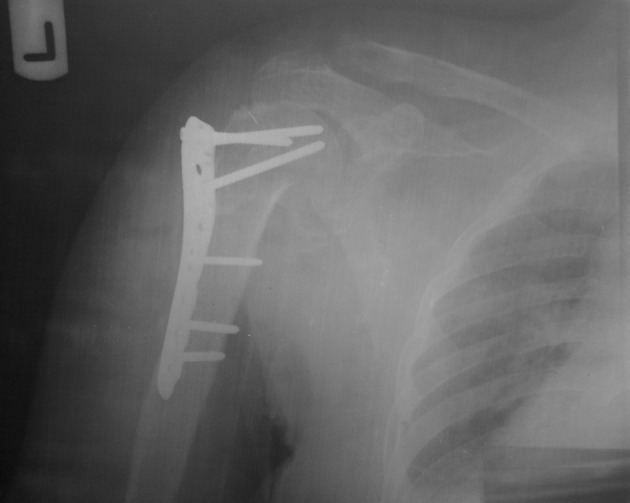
Preoperative Roentgenogram of the Left Proximal Humerus and Distal Clavicle
